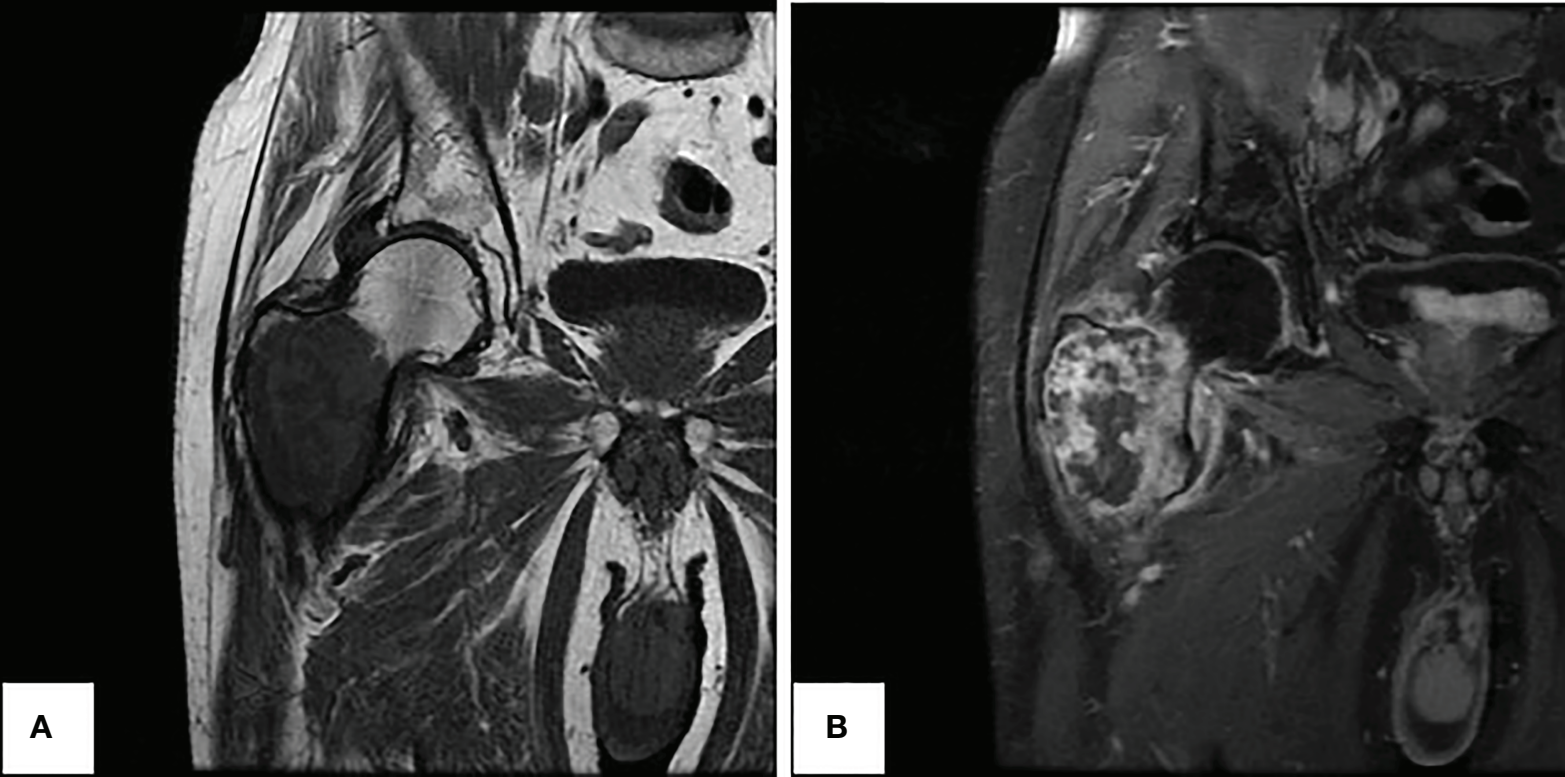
Preoperative MRI of right femur demonstrating large femoral ltyic mass. (A) Coronal view in T1 sequence (left). (B) Coronal view in T1 FAT-SAT sequence with algorithm (right).
